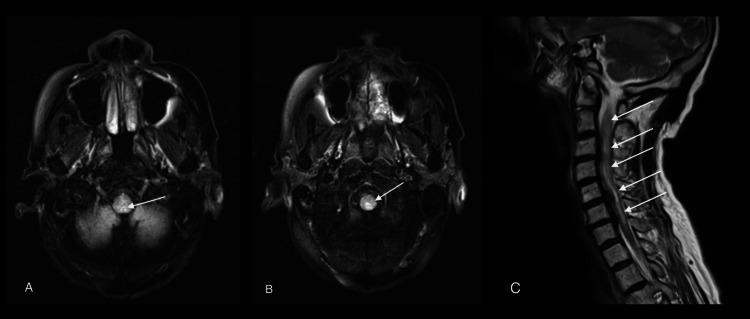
MRI T2 FLAIR brain axial and sagittal views (A) Visualisation of the lower medulla (brainstem) at the level of the cerebellum. Note a hyper-attenuated lesion situated at the dorsal medulla with increased T2 signal and mild diffuse and heterogeneous enhancement FLAIR: Fluid attenuated inversion recovery (B) Axial views of cervical cord with increased T2 signals with heterogeneous enhancement (C) Sagittal views of cervical cord with diffuse hyperintense T2 up to C7 MRI: magnetic resonance imaging; FLAIR: fluid-attenuated inversion recovery
